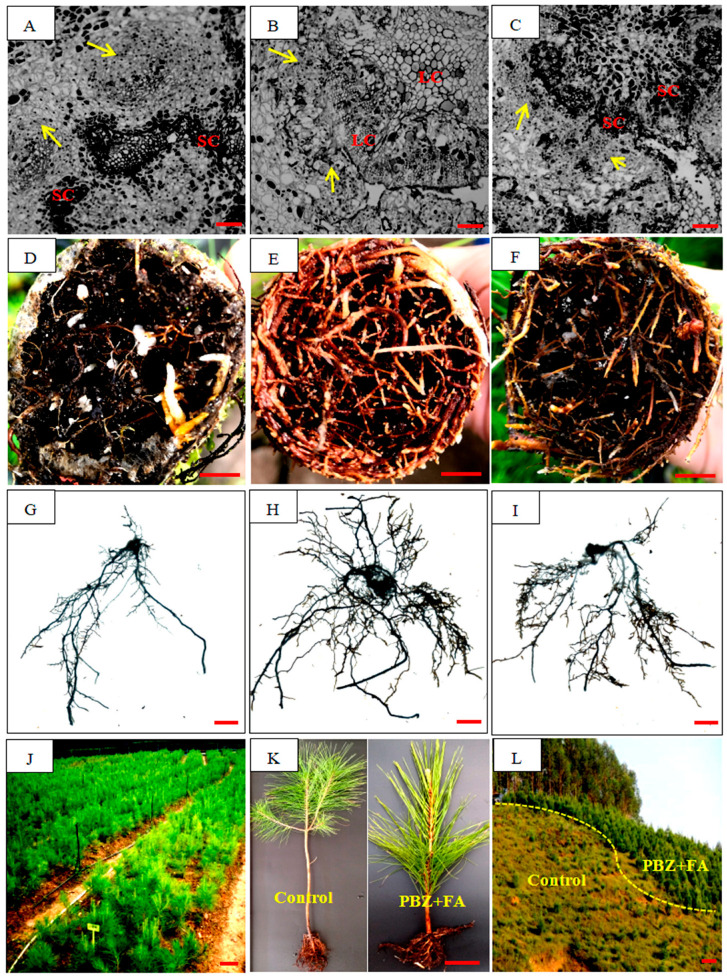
Effects of FA or FA + PBZ on rooting of *Pinus massoniana* cuttings. (A–C) anatomy of cuttings at root formation stage (cutting 35 d) under FA treatment, and the arrow shows the induced root primordium. (A) CT (200 mg·L^−1^ NAA), (B) F1 (200 mg·L^−1^ NAA + 200 mg·L^−1^ FA), (C) F2 (200 mg·L^−1^ NAA + 600 mg·L^−1^ FA); (D–I): root morphology of cuttings after 2-months cutting under FA treatment, (D,G) CT (200 mg·L^−1^ NAA), (E,H) F1 (200 mg·L^−1^ NAA + 200 mg·L^−1^ FA), (F,I) F2 (200 mg·L^−1^ NAA + 600 mg·L^−1^ FA); (J–L): Large-scale production and application of cutting seedlings through the combined use of 200 mg·L^−1^ NAA + 100 mg·L^−1^ PBZ + 200 mg·L^−1^ FA, (J) scion orchard; (K) cutting seedlings after 5 months of cutting; (L) 2-year-old clonal forest. LC, lignified cells. SC, Suberized cells. Scale bar: (A–C) 200 μm; (D–I) 1 cm; (J) 15 cm; (K) 5 cm; (L) 2 m.
